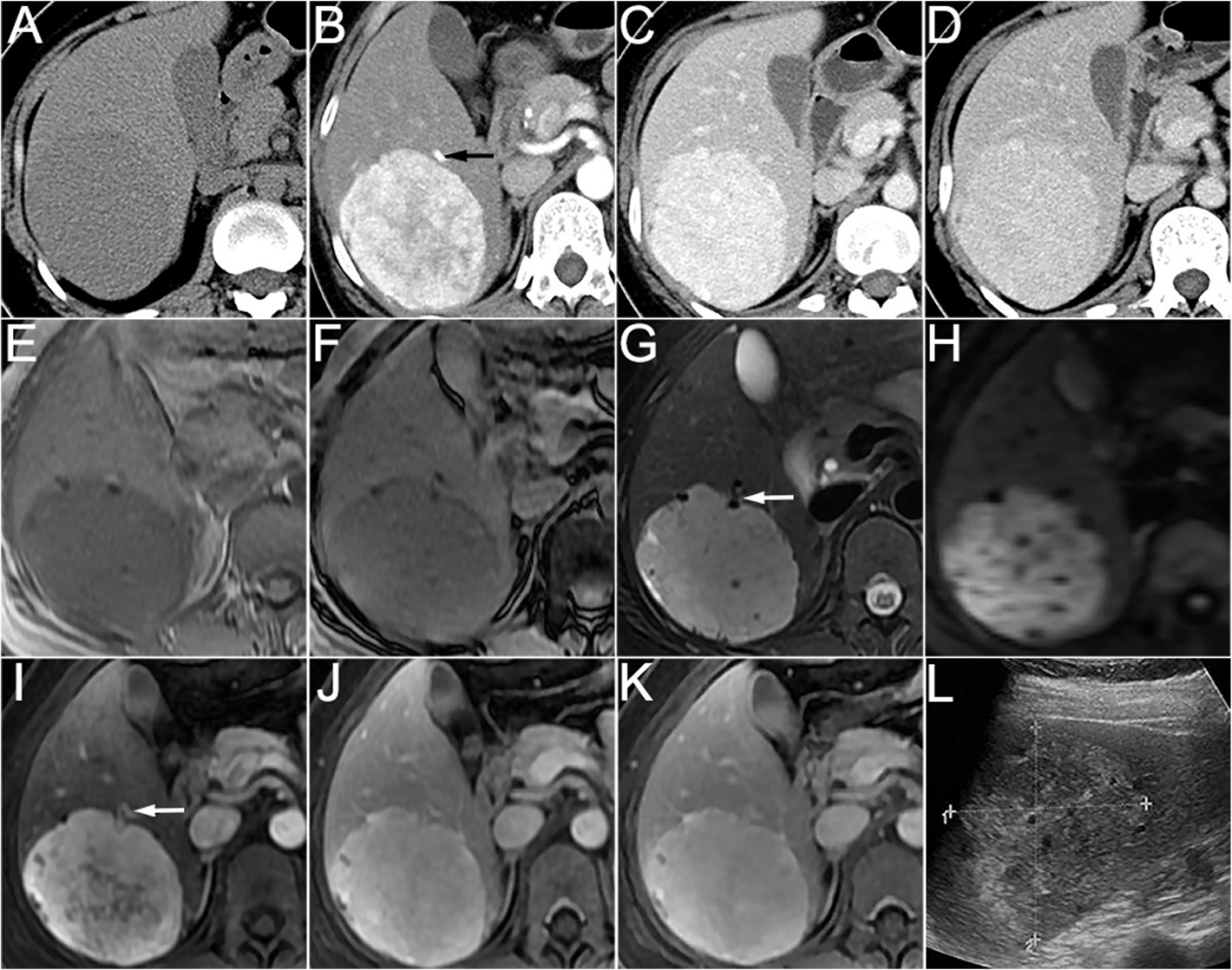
A 39-year woman with hepatic lymphangioleiomyoma. The non-enhanced CT scan (a) revealed a round well-defined homogeneous hypoattenuated mass in the right hepatic lobe. On the arterial phase (b), the mass demonstrated significantly heterogeneous enhanced surrounded by a dysmorphic vessel (arrow). On the portal venous (c) and delayed (d) phases, the mass became homogeneous, but still showed higher attenuation compared to the background liver. The MRI scan (e, T1WI in-phase; f, T1WI out-of-phase; g, fat-suppressed T2WI; h, DWI) showed hypointense on T1WI, hyperintense on T2WI and high signal intensity on DWI. The enhancement pattern on dynamic contrast-enhanced MRI (i, arterial phase; j, portal venous phase; k, delayed phase) was corresponded with CT. Note the vessel around the tumor on T2WI and arterial phase images (arrow). Ultrasound (l) showed a round, well-defined hyperechoic mass with a cribriform appearance in the right lobe of liver
